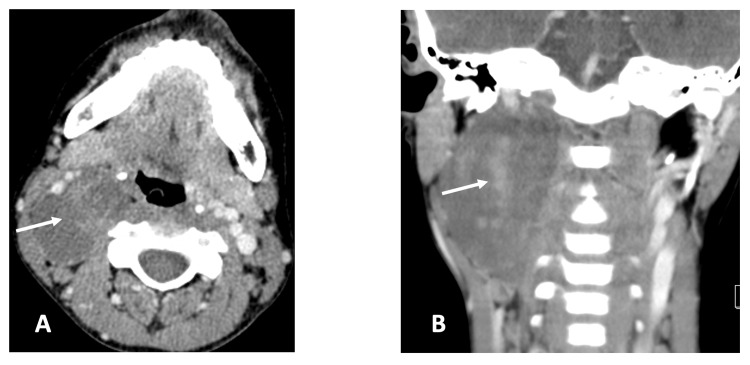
Axial (A) and coronal (B) views of contrast-enhanced CT images demonstrating a mass (white arrow) occupying the right parapharyngeal space.
